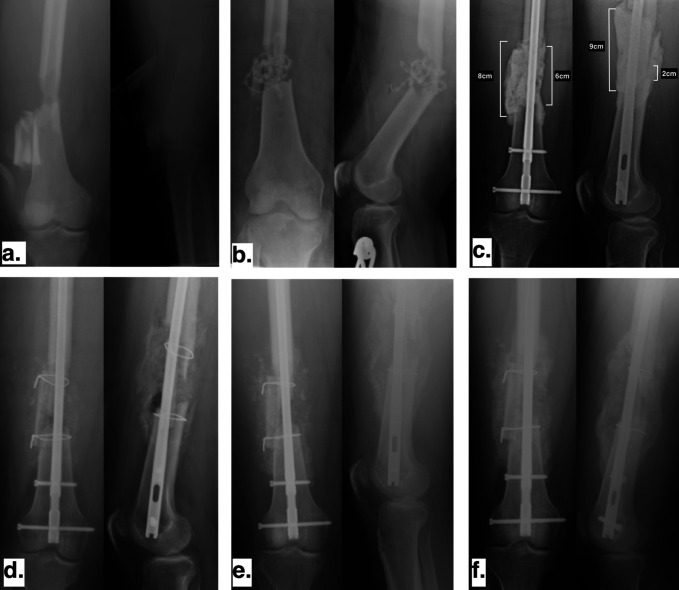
Case 2. (A) Injury radiograph. (B) Radiograph showing initial irrigation and debridement with the placement of antibiotic beads. (C) Radiograpg showing stage 1 of the induced membrane technique and fixation with intramedullary nail. (D) Radiograpg showing stage 2 of the induced membrane technique with placement of fibular allograft strut posterolaterally. (E) Radiographs at the two-week follow-up. (F) Radiographs at the 6-month follow-up.
